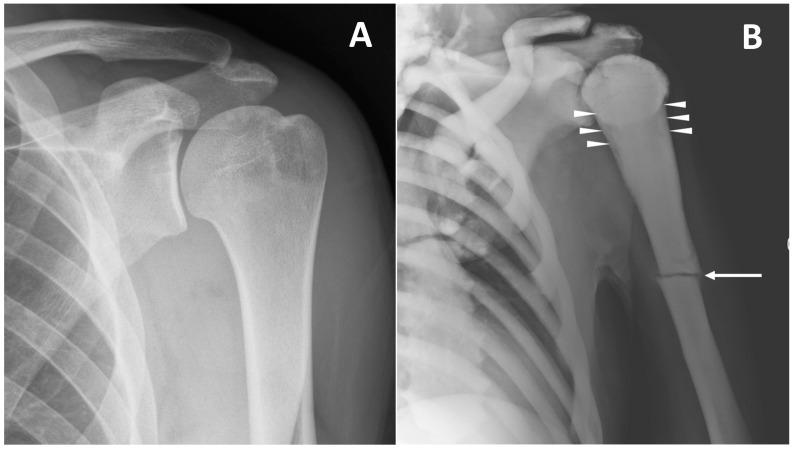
(A) conventional radiography of the left shoulder in a young healthy male (shown as comparison). (B) conventional radiography of a 45-year-old male with ARO type of osteopetrosis presenting with atraumatic pathological fracture of the diaphysis of the left humerus (arrow). A mild alteration of proximal metaphysis shape (arrowheads), and a diffuse increase in bone density of all the skeletal sites included can also be detected.
